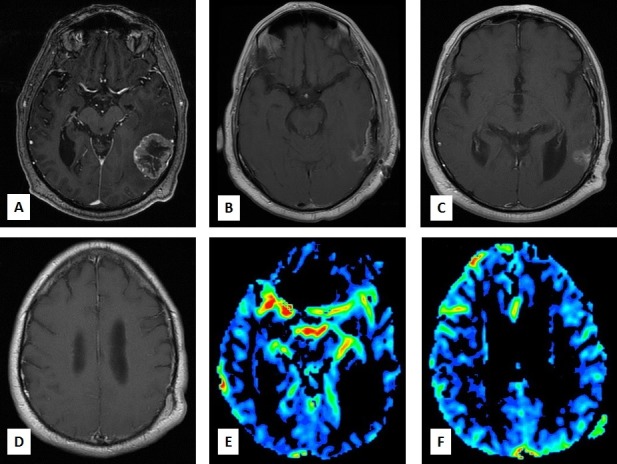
Scalp Metastasis Post Craniotomy and Radiosurgery A-D: Gadolinium enhanced T1 MRI sequences. A solitary metastatic lesion in the left parietal lobe is evident in A. B shows post-surgical changes following craniotomy. Enhancement in the surgical cavity is seen (C), and a new lesion in the left parietal bone is noted (D). E-F: MR perfusion images showing no blood flow in the surgical cavity (E), and increased blood flow in the region of scalp metastasis (F).
